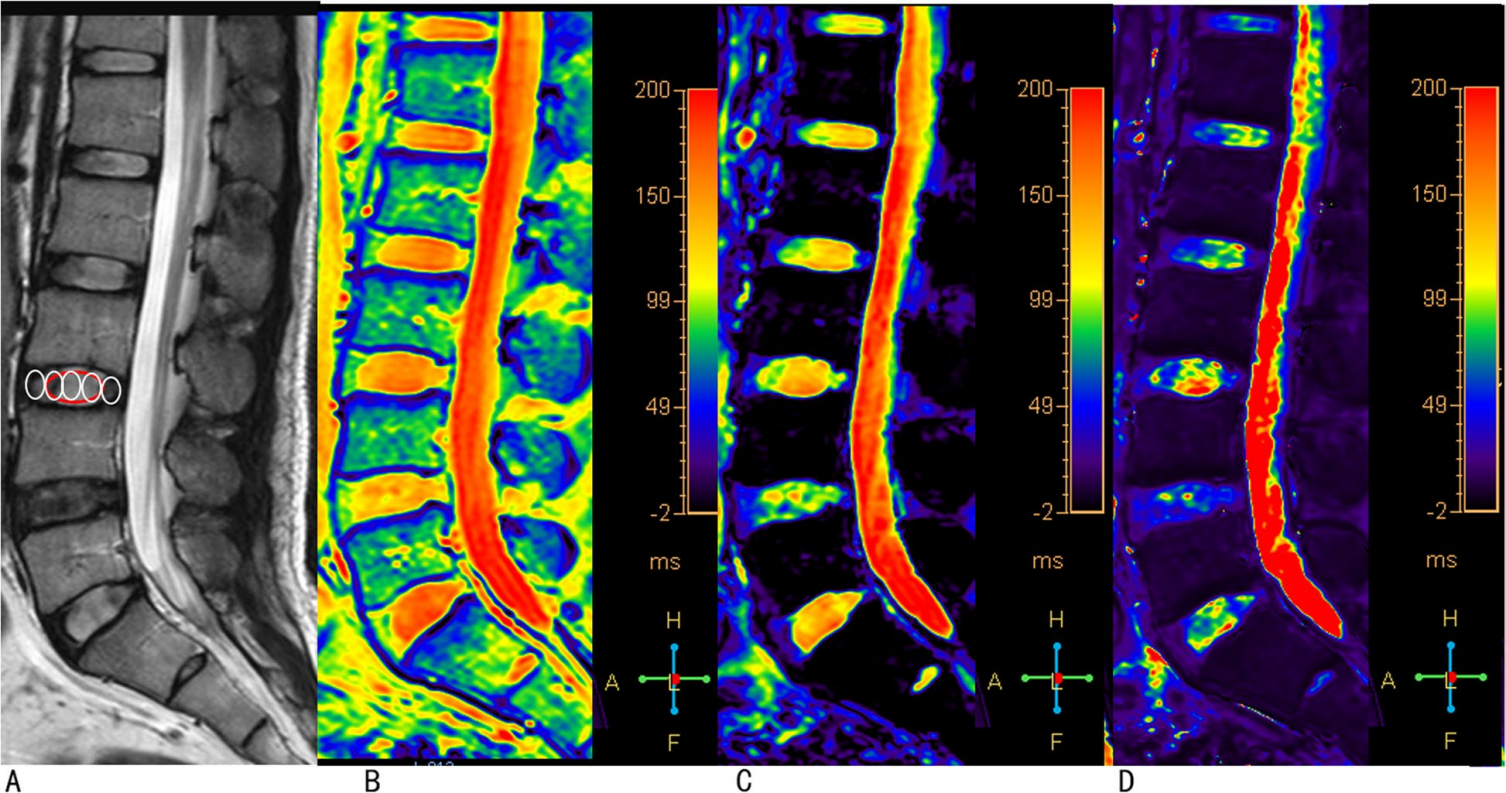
Representative images of sagittal T2-weighted images, T1ρ map, T2 map and T2* map. Five oval regions-of-interest (ROIs) were firstly placed on the T2-weighted images (A), then the ROIs were transferred to the T1ρ map (B), T2 map (C), and T2* map (D). The central three ovals (included in one red oval) represent nucleus pulposus, and the ventral and dorsal one represents anterior annulus fibrosus and posterior annulus fibrosus, respectively
